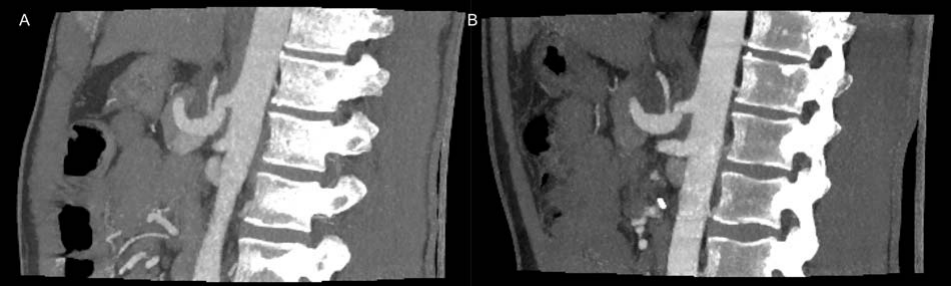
Postoperative inspiratory (A) and expiratory (B) computed tomography scan with repealed hook formation as well as stenosis of the celiac artery.
